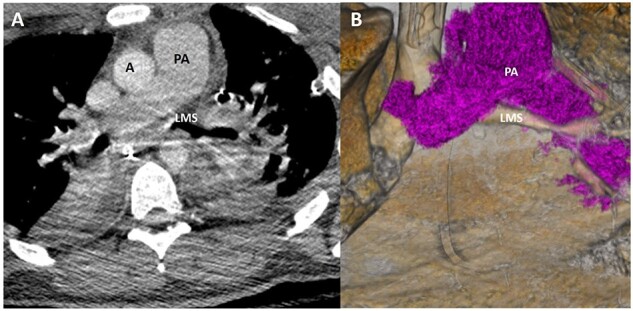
Representative image of axial slice of CT scan (A) and coronal 3D reconstruction (B) on HD 4 showed marked improvement in LMS patency.
